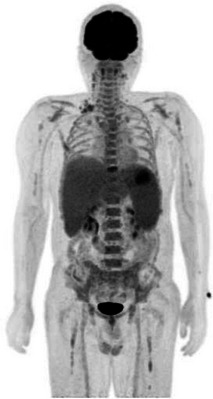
The diagnostic FDG‐PET scans of the patient.
